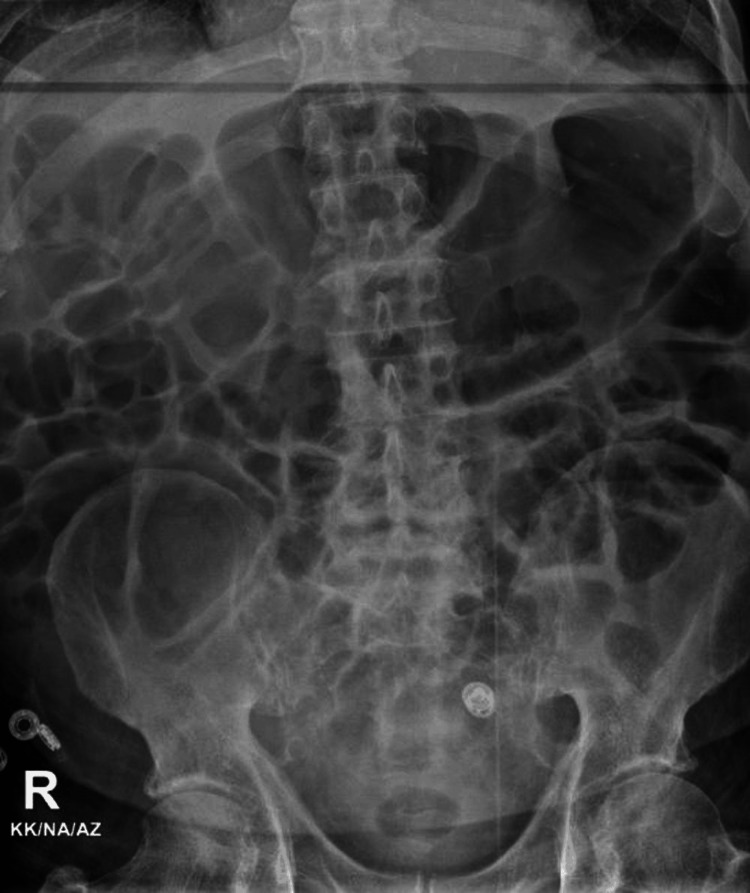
Abdominal X-ray showing a non-specific bowel gas pattern.
